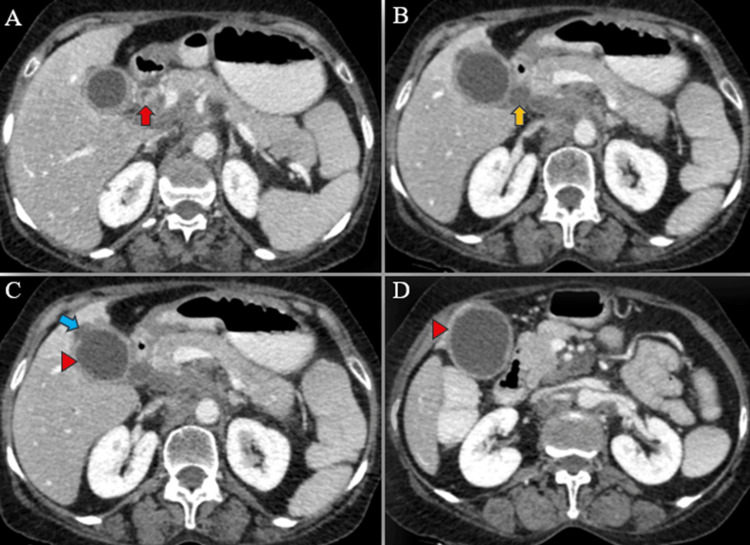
Post-contrast axial CT images reveal circumferential thickening of the mid-CBD (red arrow) (A) along with dilation of the proximal CBD (yellow arrow) (B). Additionally, a defect in the gallbladder body region (blue arrow) (C) is accompanied by a surrounding hypodense pericholecystic collection and thickened gallbladder wall (red arrowheads) (C-D). CT: Computed tomography; CBD: common bile duct
